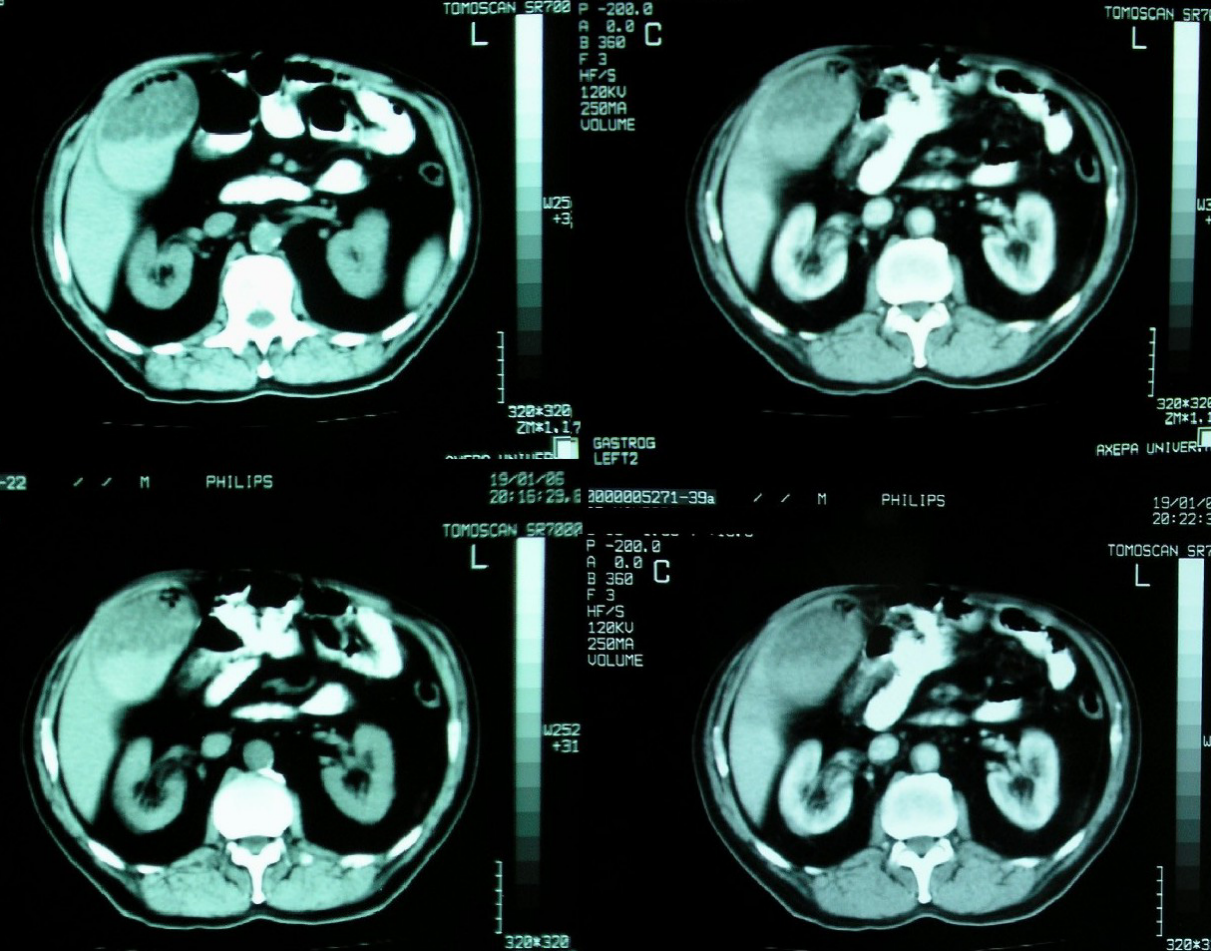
Axial sections of single slice CT imaging (section thickness 10 mm), revealed emphysematous cholecystitis with thickening of gallbladder wall and wall enhancement after iv contrast enhancement, as well as, dilatation of the gallbladder with multiple gallstones precipitate and intraluminal air.
